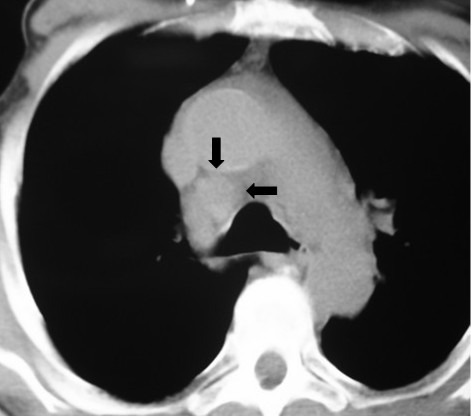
A computed tomography scan of the chest showing enlarged paratracheal lymph nodes over the superior anterior mediastinum.
